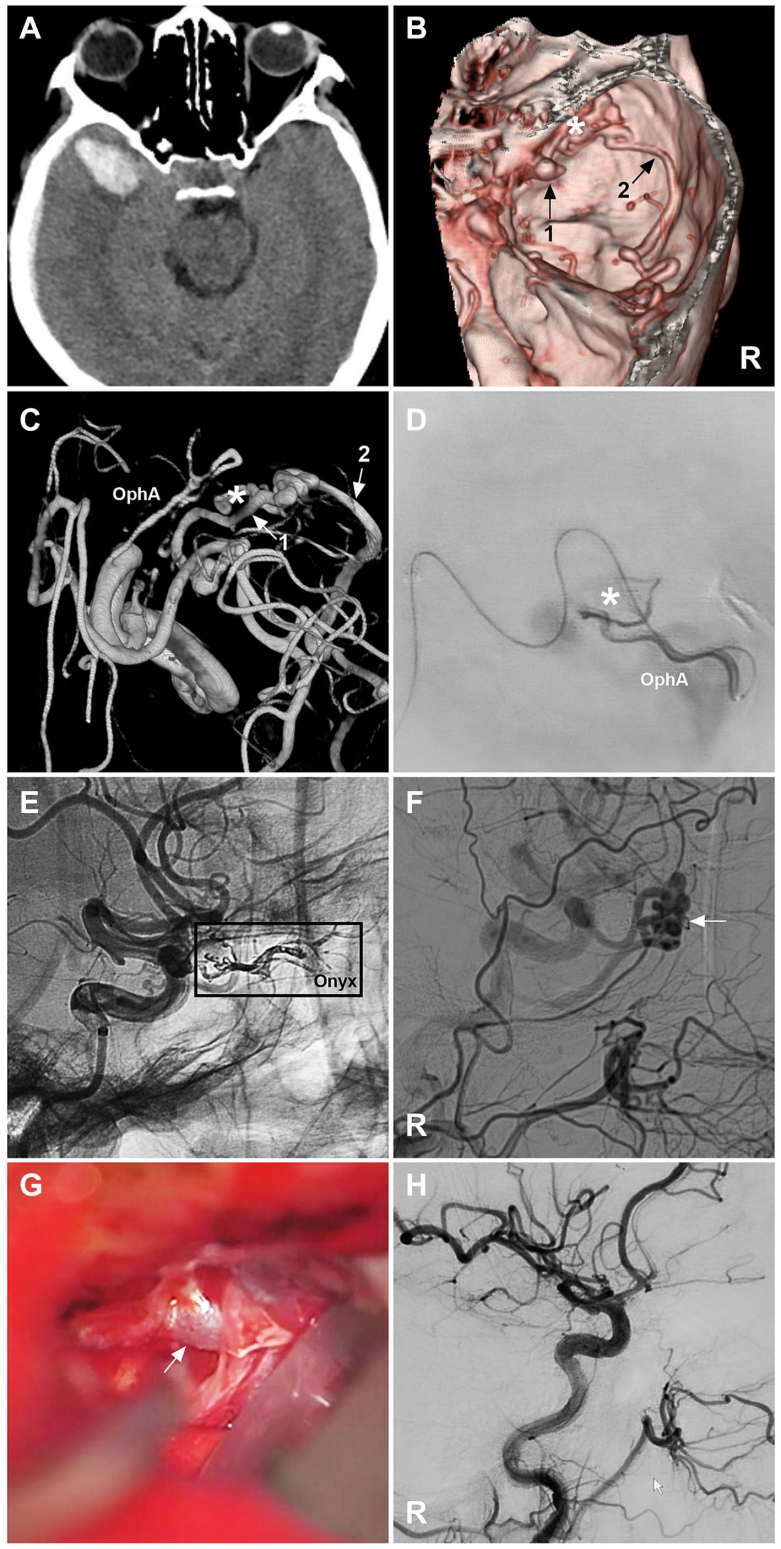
Open surgery after incomplete EVT for a sphenoidal region DAVF. (A) CT image showing a right temporal lobe hematoma. (B) CTA image showing a right sphenoidal lesser wing DAVF (asterisk); numbers 1 and 2 with arrows indicate venous drainage. (C) Three-dimensional DSA image showing that the DAVF was supplied by the recurrent meningeal branch of the OphA; the numbers 1 and 2 with arrows indicate venous drainage. (D) Selective angiography showing the DAVF (asterisk). (E) Unsubtracted DSA showing Onyx casting (frame) via the recurrent meningeal branch of the OphA. (F) DSA image showing the residual DAVF (arrow). (G) Intraoperative image of an open surgery showing the arterialized draining vein (arrow); then, the vein was coagulated and cut. (H) Immediate DSA showing that the DAVF was obliterated. CT, computed tomography; CTA, computed tomography angiography; DAVF, dural arteriovenous fistula; DSA, digital subtraction angiography; EVT, endovascular treatment; R, right; OphA, ophthalmic artery. After EVT, the patient suffered right eye blindness.
